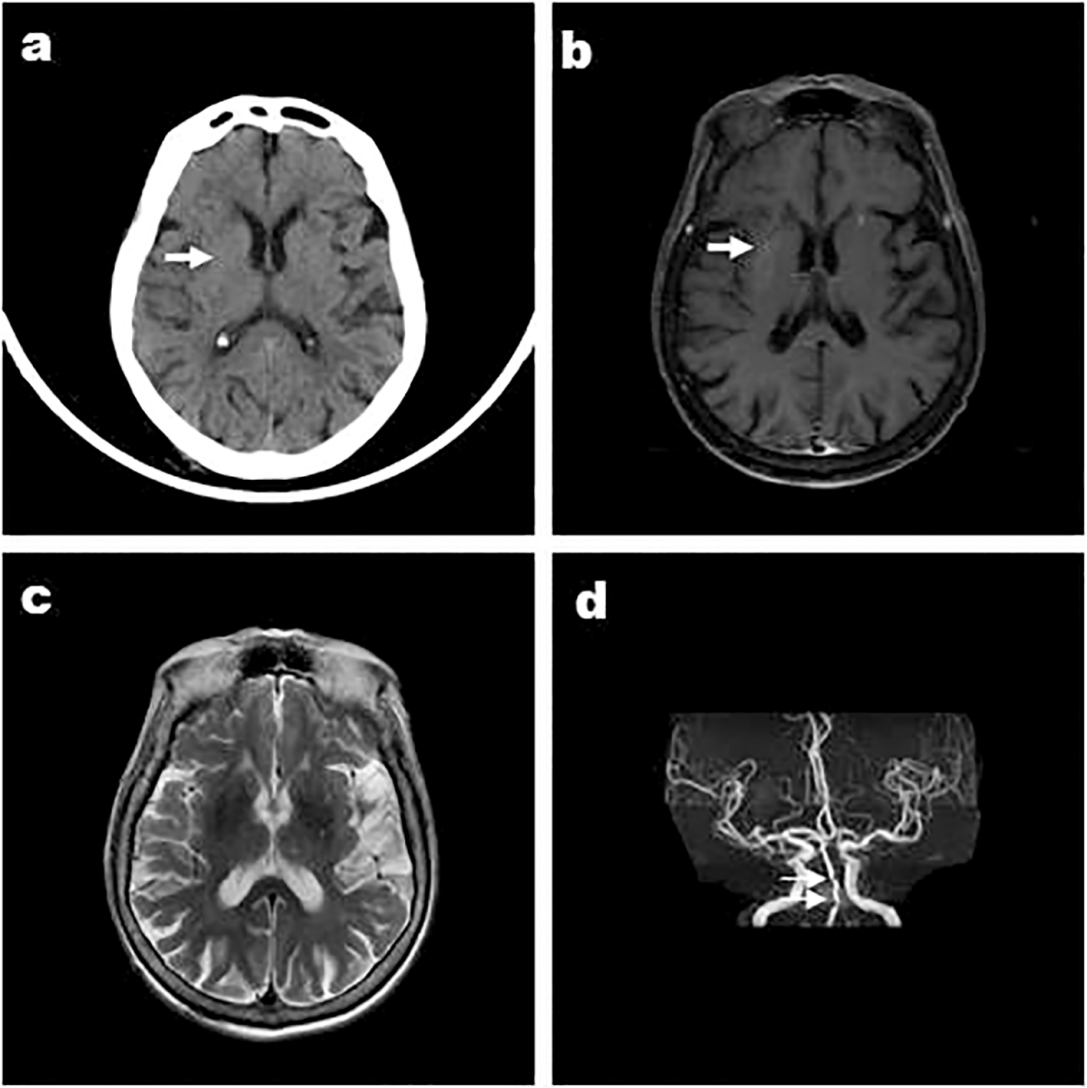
Case 4, an 88-year-old female, was admitted to hospital with “involuntary movements of the left limb for 15 days” and images showed that the right striatum of involuntary movements was high density on CT (A), high signal on T1WI (B) Arrows indicate arterial stenosis, iso-signal on T2WI (C), and MRA showed arterial stenosis (D). Arrows indicate bilateral striatum abnormal signal.
